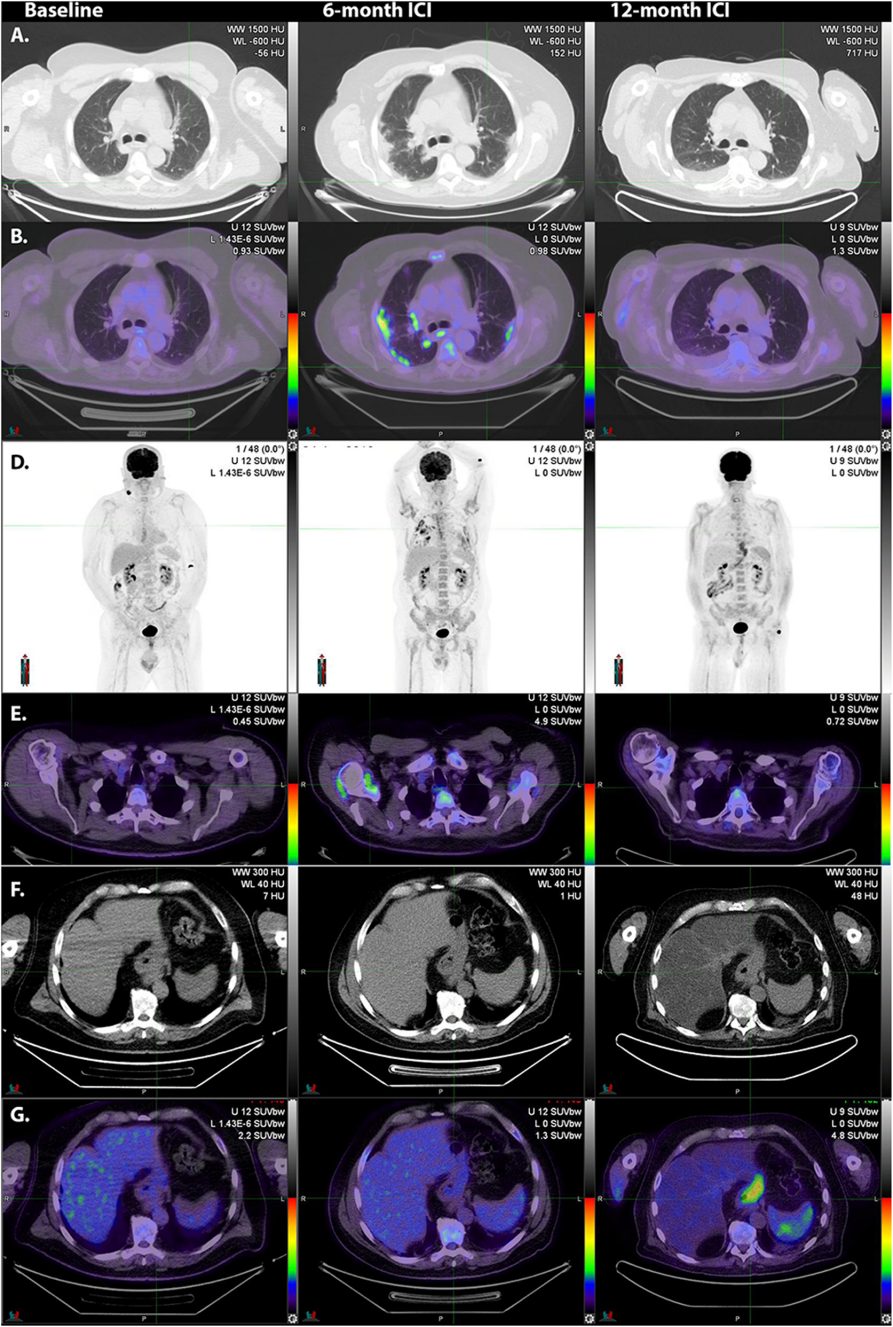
Following resection of nodal metastasis in the right lower cervical region, adjuvant immune checkpoint inhibitor therapy was commenced. At 6-month surveillance ^18^F-FDG PET/CT, lung infiltrates were apparent on correlative CT (A). These had high ^18^F-FDG -avidity (B, middle panel). Serial MIP images (C) demonstrate the patchy, bilateral nature of these changes as well as generalized bone marrow activation and increased activity in both shoulders consistent with synovitis as confirmed on transaxial fused ^18^F-FDG PET/CT images (D, middle panel). With the introduction of corticosteroids, the pneumonitis resolved but a fatty change was noted in the liver (F, right panel) and diffuse oesophagogastric uptake was observed (G, right panel). Gastroscopy confirmed enteritis. Increased spleen to liver ratio on the late scan likely reflects a combination of reduced hepatic activity related to steatosis and splenic activation
